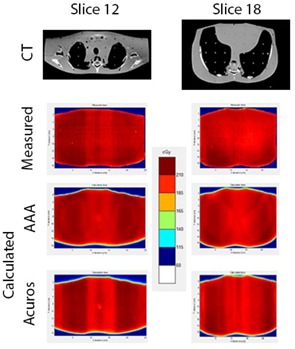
CT images of slices 12 and 18, along with the measured film dose and AAA and Acuros calculated dose distributions.
